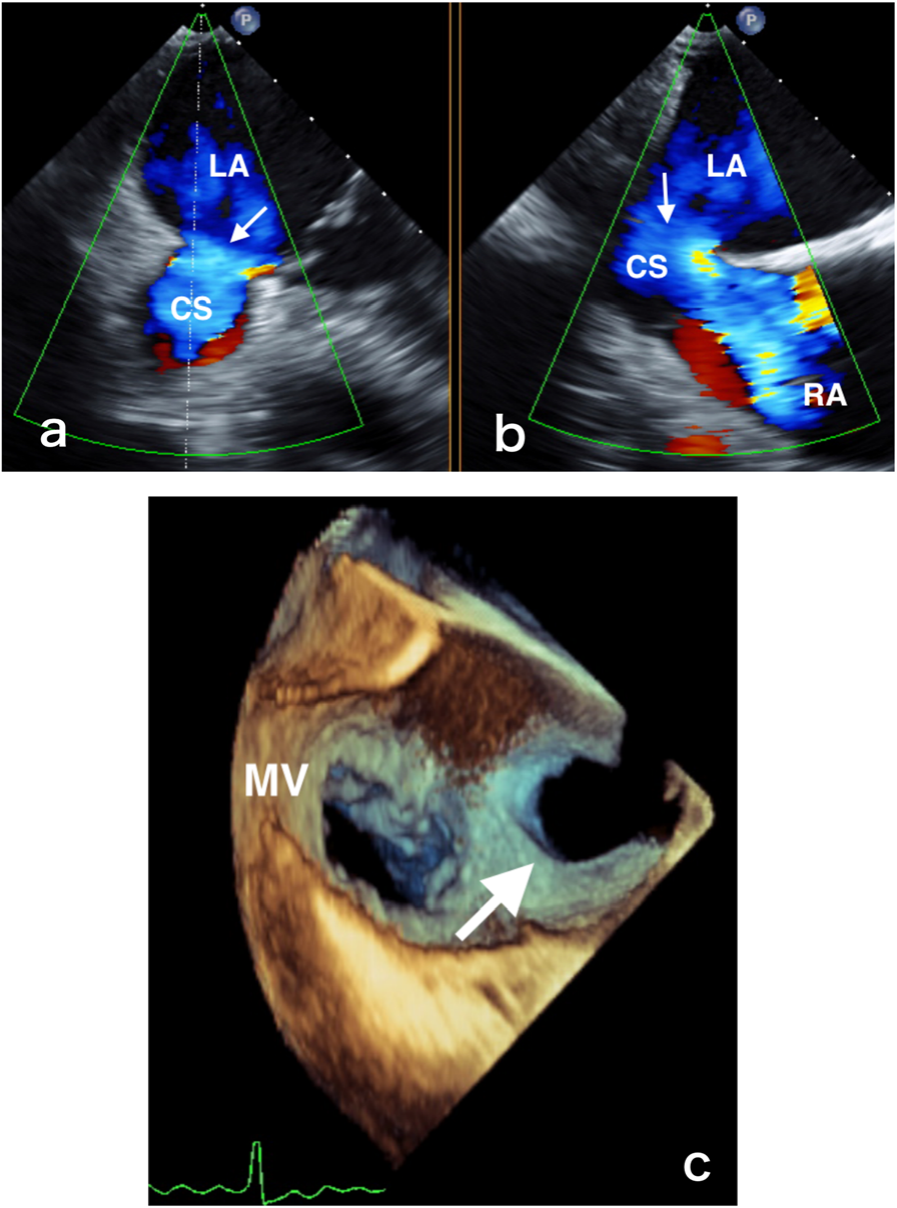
Echocardiographic findings in cases 3 and 5. a, b Dynamic left-to-right shunting visible through the defect in case 3. c Three-dimensional transesophageal echocardiography in case 5 clearly showing a defect (white arrow) near the posterolateral commissure of the mitral valve. *CS* coronary sinus, *LA* left atrium, *MV* mitral valve, *RA* right atrium
